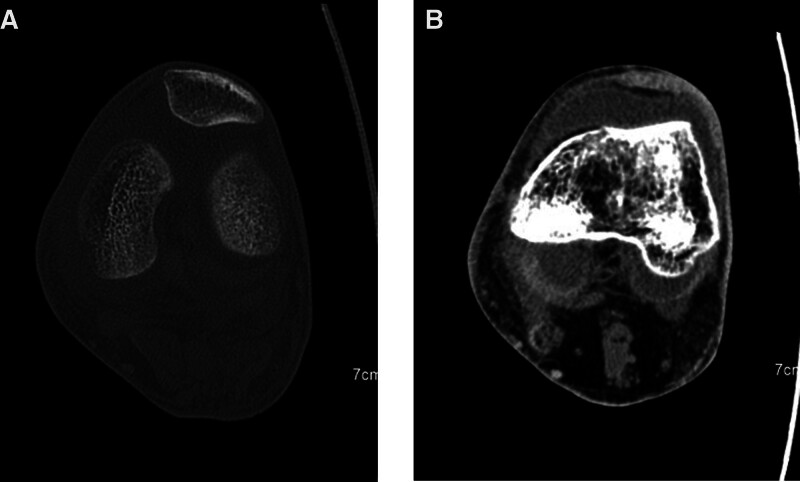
Knee-joint lesions in case 2 of CT scan. (A) Bone destruction seen in the bone window. (B) Fluid effusion and surrounding soft tissue swelling seen in the soft tissue window.
